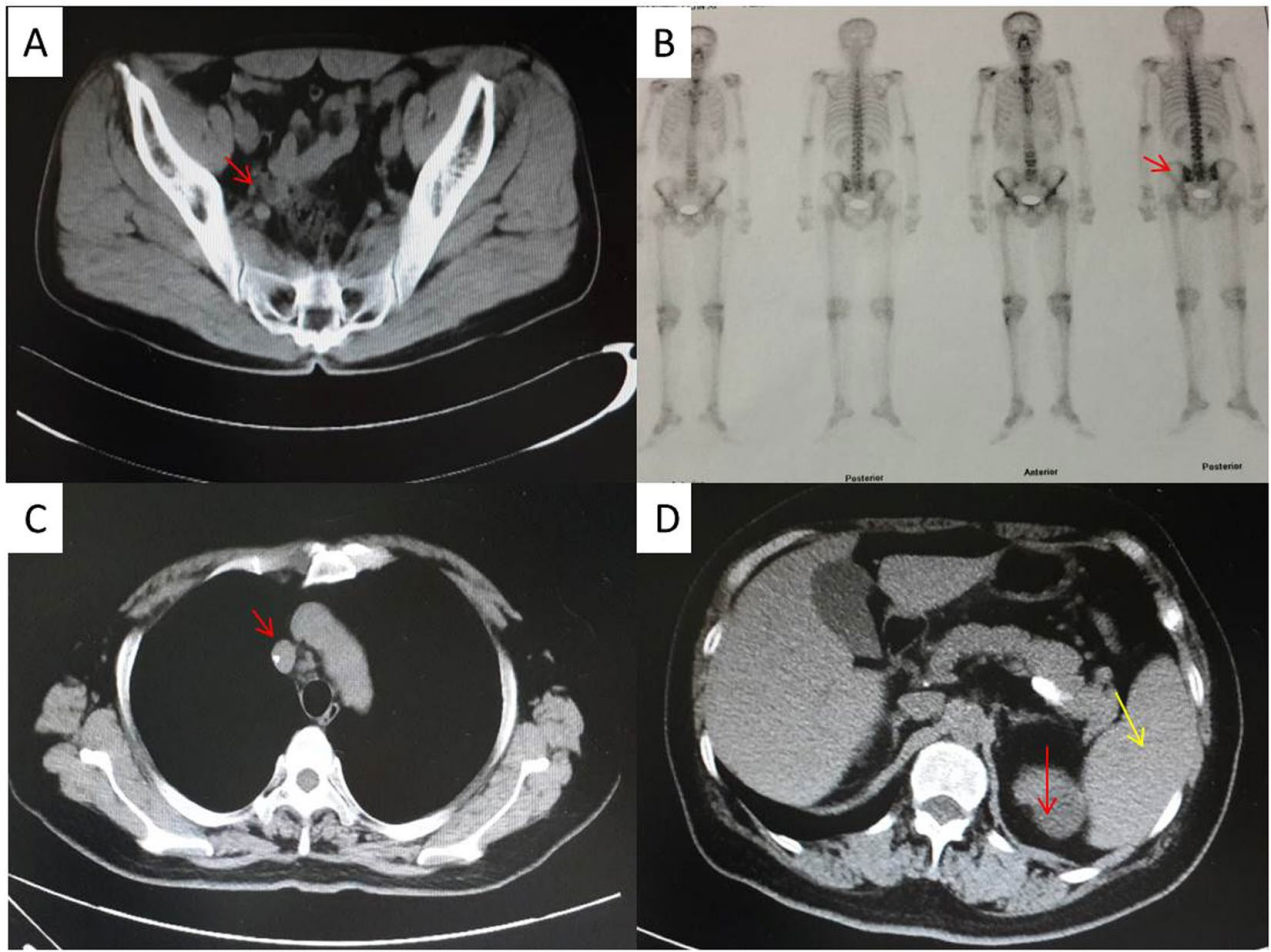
Pelvic CT scanning in case 1 displayed the lymph nodes aside the left external iliac blood vessels enlarged, with the biggest size of 1.8 × 1.4 cm (A). PETCT in case 1 showed a high bone salt metabolism in right coracoid and left sacroiliac joint (B). Chest CT scanning in case 2 displayed multiple lymph nodes enlarged in right hilar and mediastinal (C). Abdomen CT scanning in case 2 displayed a clear borderline mass in the right kidney (red arrow) and a slightly larger spleen (yellow arrow) (D).
